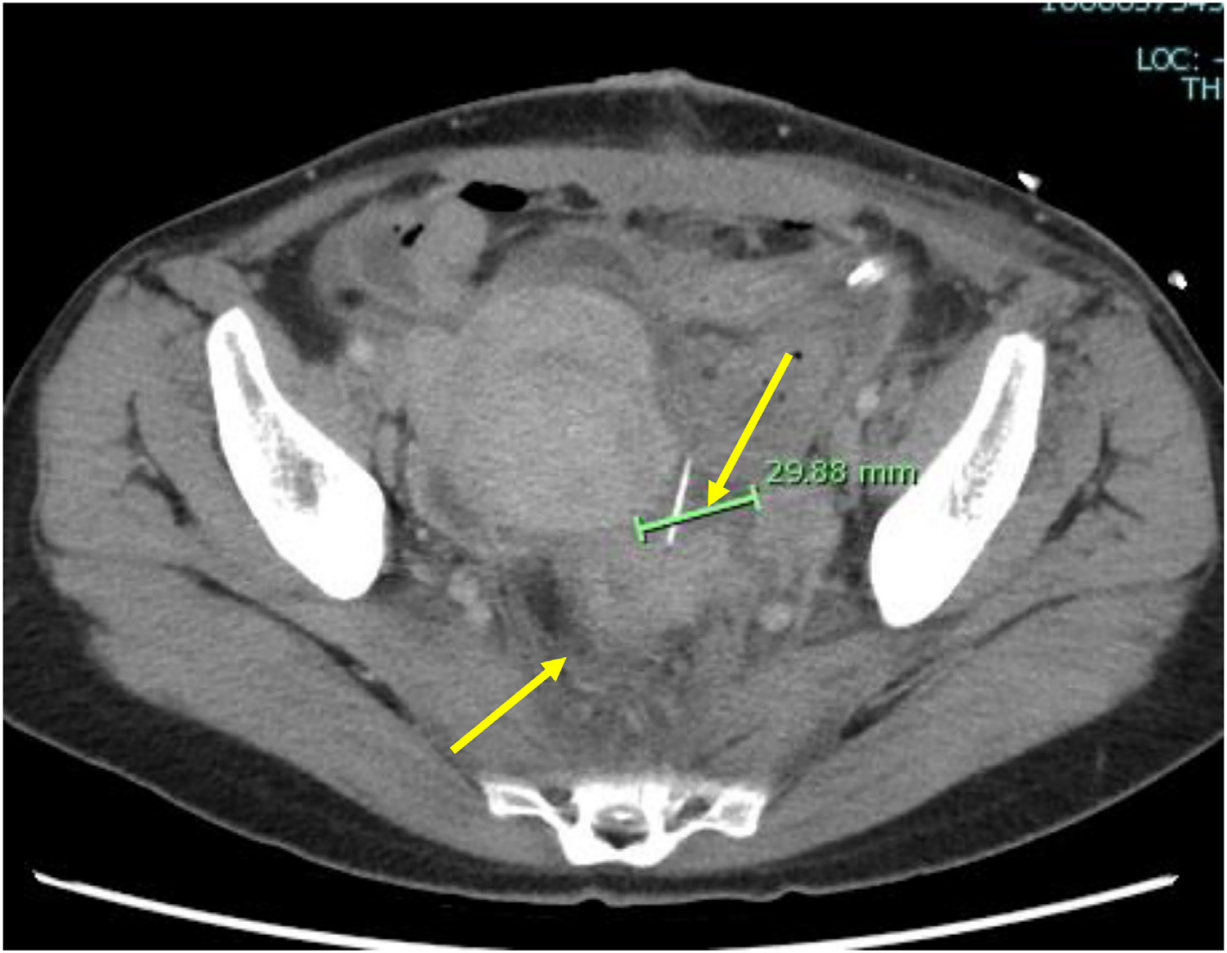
CT performed on the fourth postoperative date: yellow arrow indicating the abscess and surgical drain.
